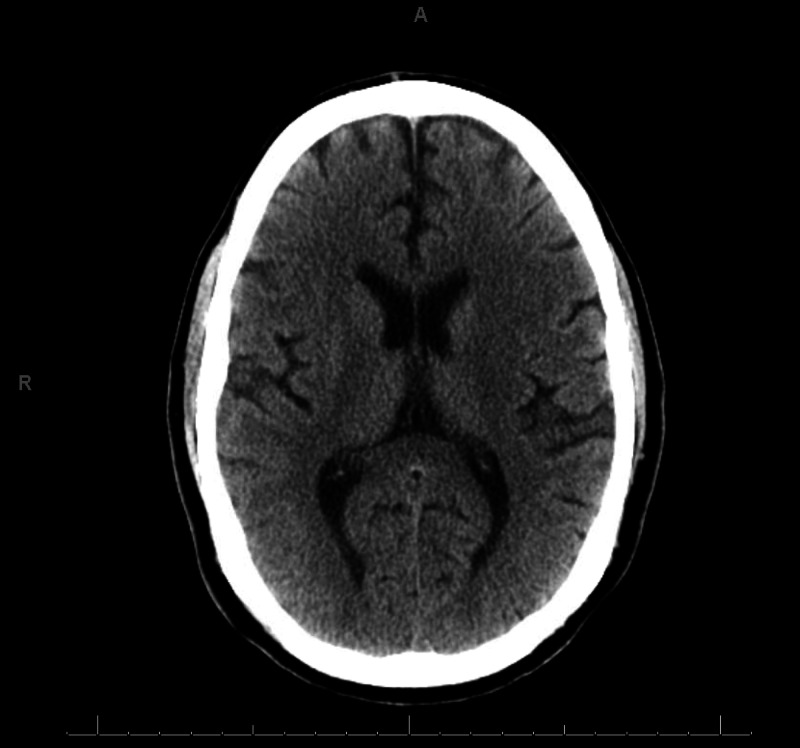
Head CT of the Patient Non-contrast CT of the brain demonstrates no evidence of intracranial pathology that would suggest a structural cause for the patient's decrease level of consciousness.
